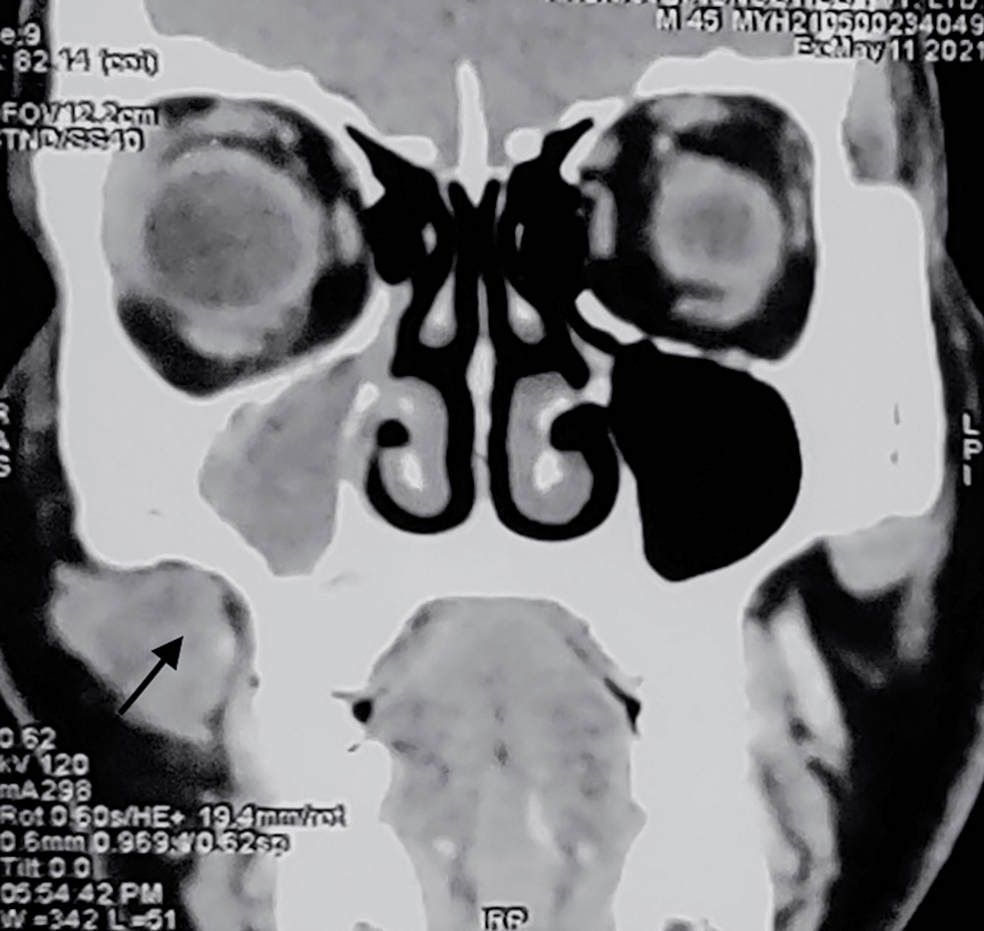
CT coronal section showing mildly enhancing soft tissue in the pre-maxillary region. CT: computed tomography
